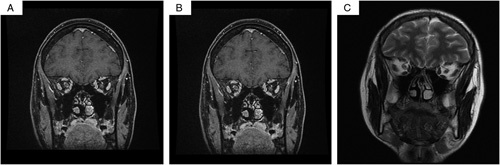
(A) MRI of the brain with a coronal section with no contrast done on 1.5 T showed enhanced swollen left optic nerve with high T2-signal and perioptic fat stranding compared to the normal left side, suggestive of optic neuritis. (B) MRI with a coronal section of the brain with no contrast done on 1.5 T showed enhanced swollen left optic nerve with high T2-signal and perioptic fat stranding compared to the normal left side, suggestive of optic neuritis. (C) MRI of the brain with a coronal section with no contrast done on 1.5 T showed enhanced swollen left optic nerve with high T2-signal and perioptic fat stranding compared to the normal left side, suggestive of optic neuritis.
